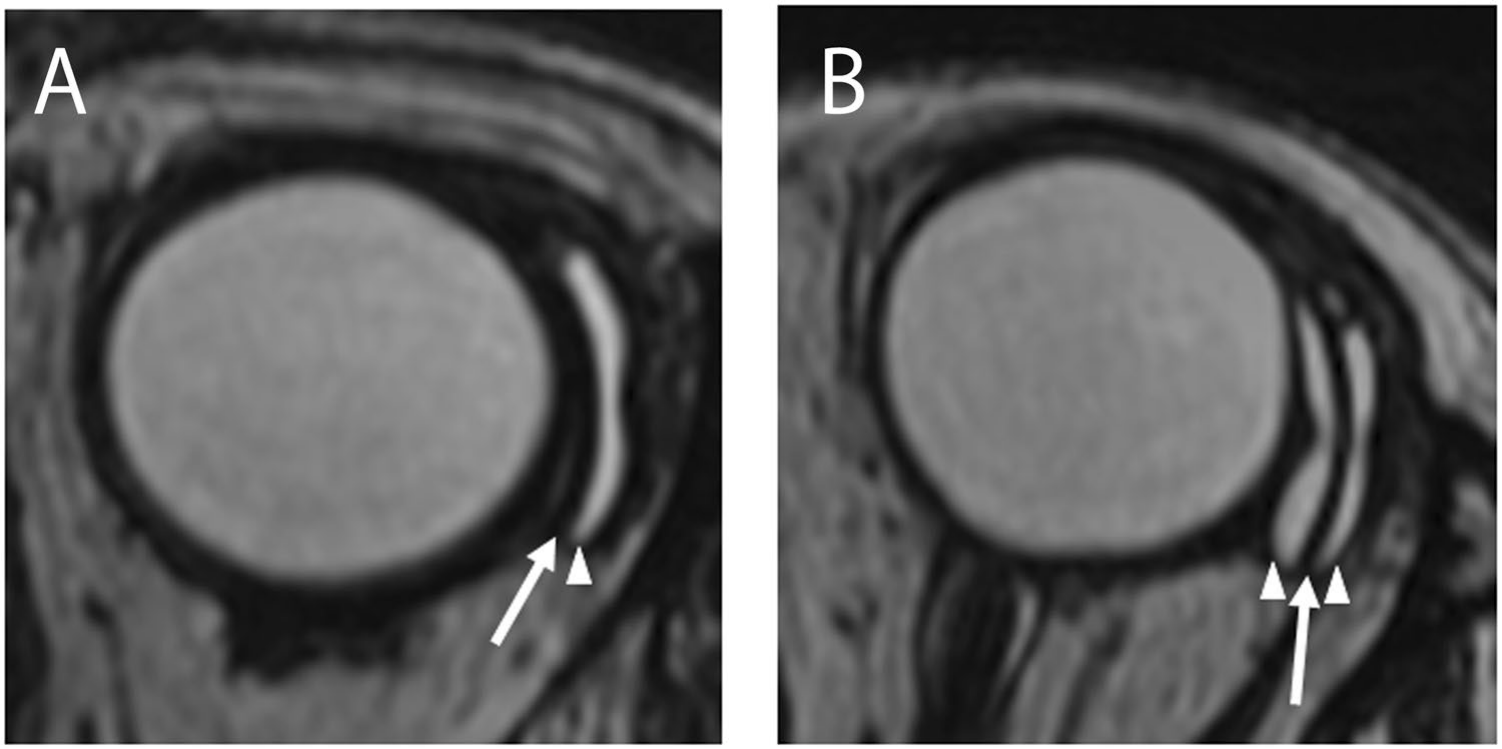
Magnetic resonance imaging scans of eyes with the Baerveldt glaucoma implant. (A) A case with a single bleb layer. (B) A case with a double bleb layer. A curvilinear low-intensity area was observed at the location of the endplate (arrows). A high-intensity area corresponding to the signal intensity of the aqueous humor, was located adjacent to the endplate (arrowheads).
